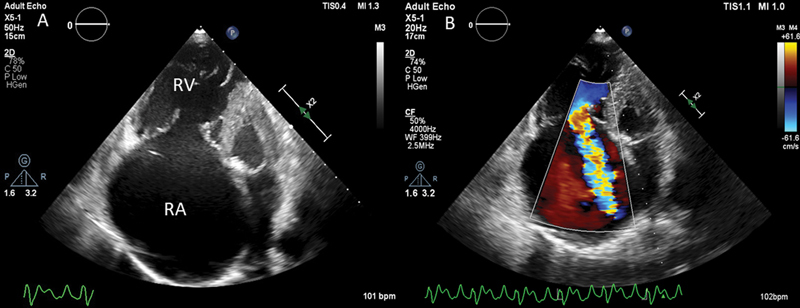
Echocardiogram in modified apical four-chamber view revealing dilated RA and RV (
